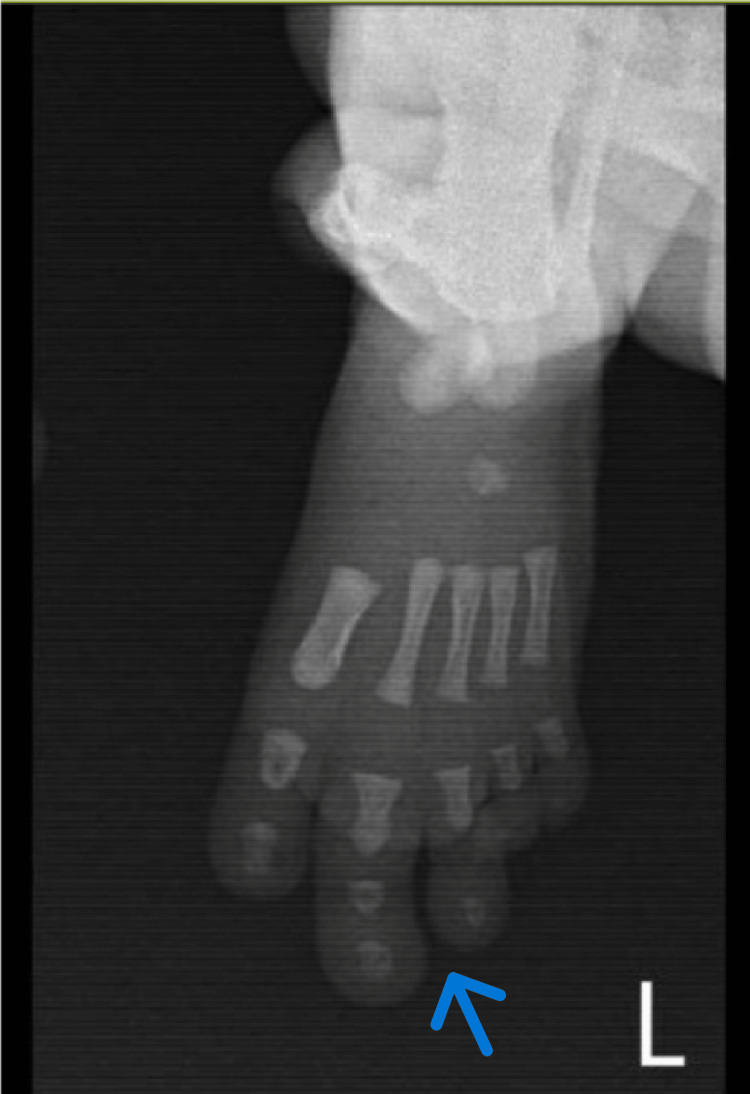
Radiograph of the left foot; enlargement of the soft tissue and bone.
